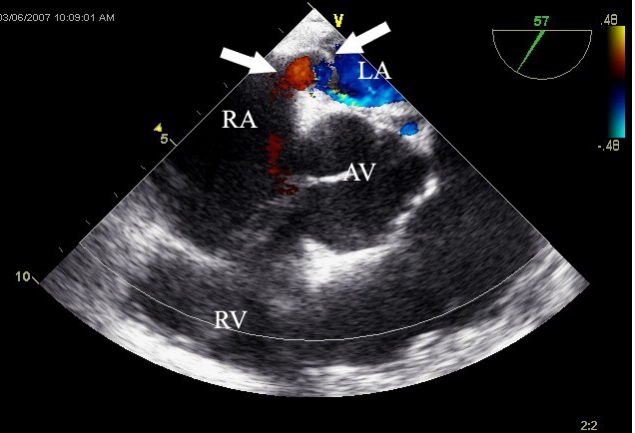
Echocardiogram shows the presence of a large PFO.
